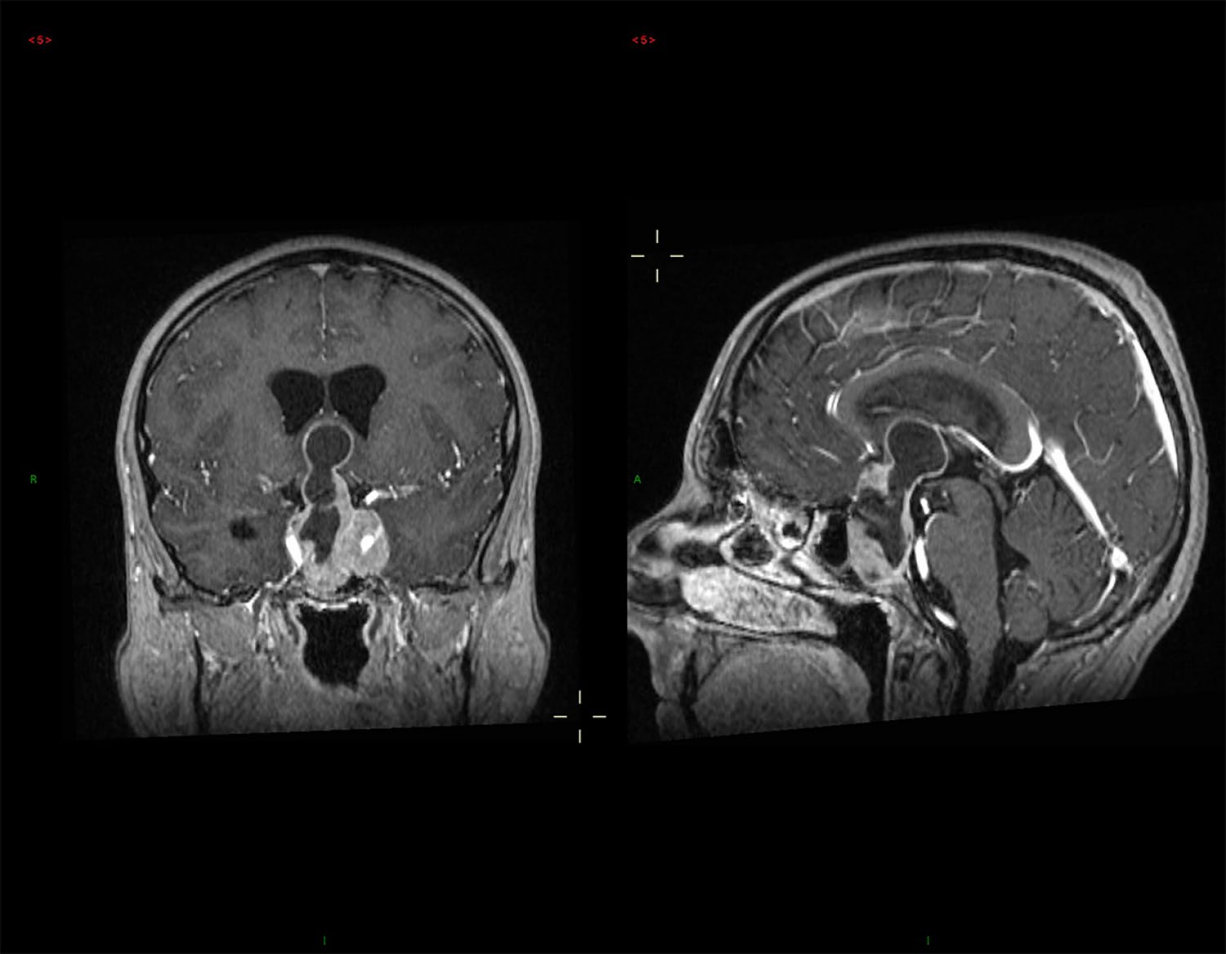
Coronal (left) and sagittal (right) MRI images pre treatment
